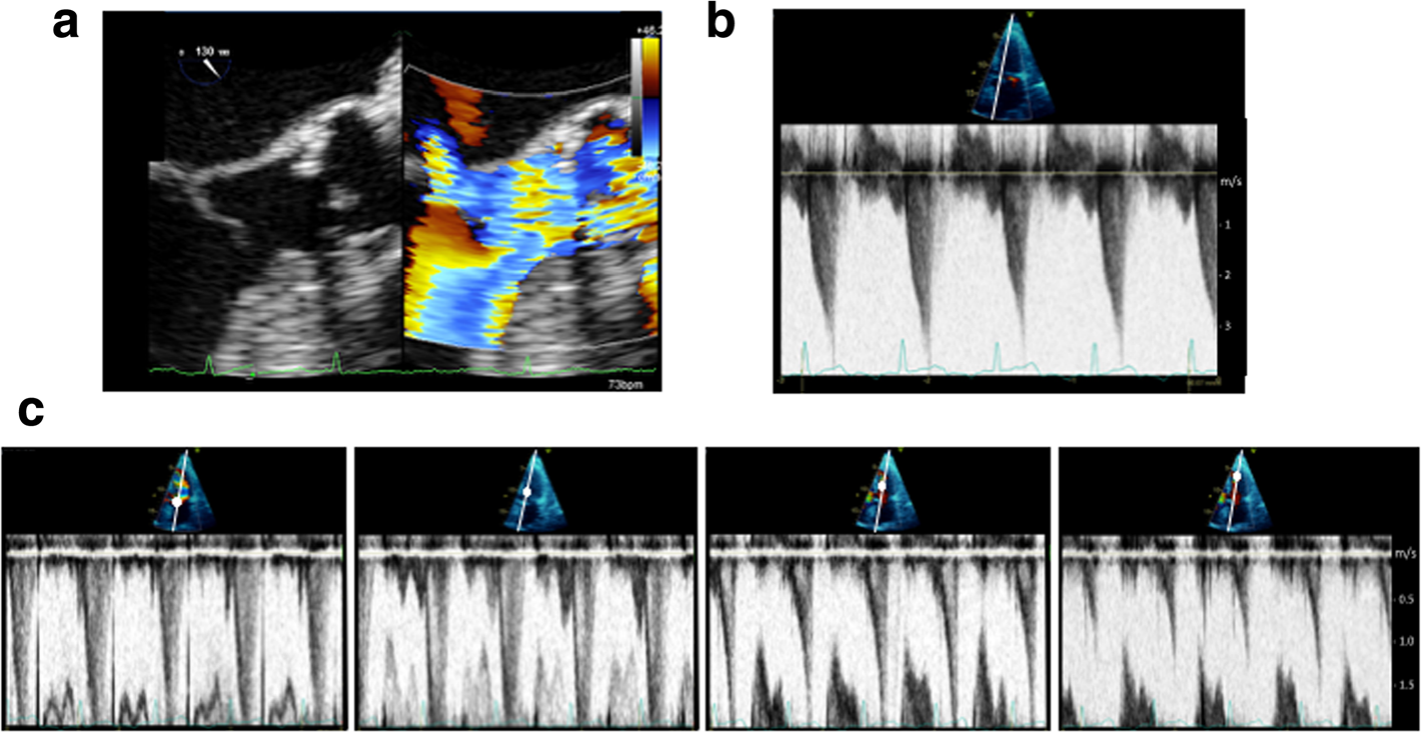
Left ventricular cavity obstruction. Obstruction of the left ventricular cavity can be due to anatomical factors and/or precipitated by LV cavity obstruction from hypovolaemia, excessive inotropic agents, predisposing anatomical abnormalities, etc., a systolic anterior motion of theanteiror mitral valve leafelet is frequently seen, often with resulting mitral regurgitation. Accurate imaging to determine location of the obstruction is required. b Continuous wave Doppler can identify an obstruction through recognition of the classic ‘dagger’ shaped curve with its peak in late systole. c Pulsed wave Doppler can identify the point of restriction by sequentially moving the gate from the left ventricle outflow tract to the apex looking for the point of maximal flow (note that aliasing is frequently seen where the maximum gradient is too high for the pulsed wave Doppler scale)
